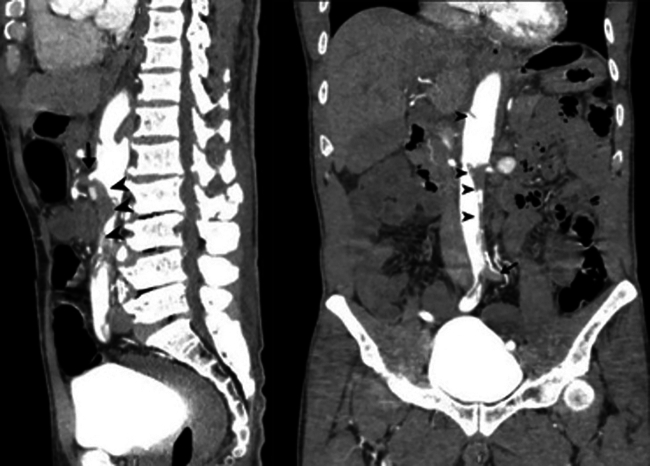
Abdominal aortic injury with superior mesenteric artery (SMA) occlusion in multi-detector computed tomography (*arrowhead*, aortic dissection with multiple entry; *arrow*, SMA total occlusion).
